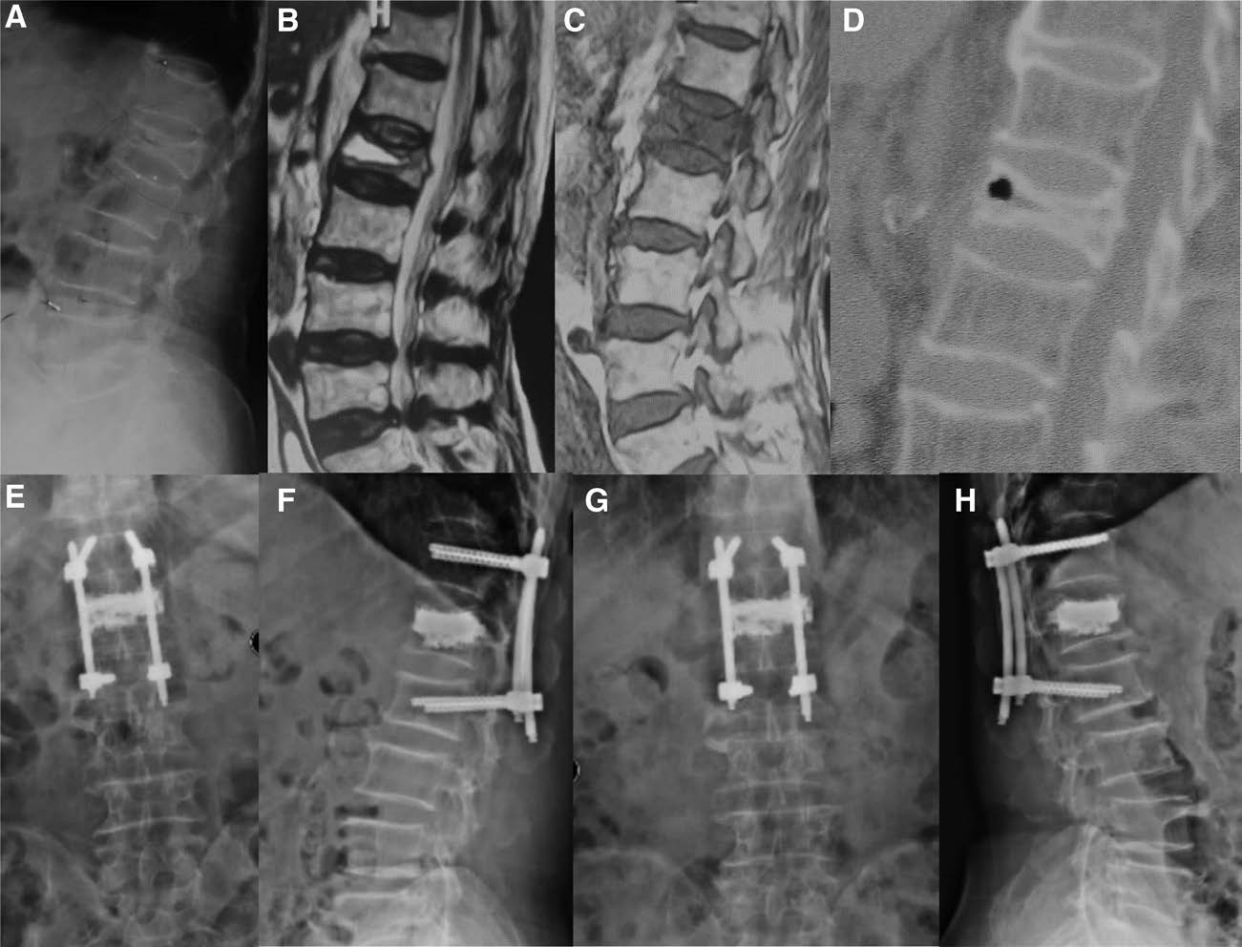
A 76-year-old-male patient who underwent percutaneous fixation combined with vertebroplasty for L1 Kümmell disease. (A–D) Preoperative imaging showed L1 vertebral fracture with “vertebral body vacuum fissure” sign. (E and F) Postoperative X-ray showed fine bone cement position and injury vertebral body height recovery. (G and H) Postoperatively 12 months X-ray showed firm internal fixation, stable vertebral body height, and fine bone cement position.
